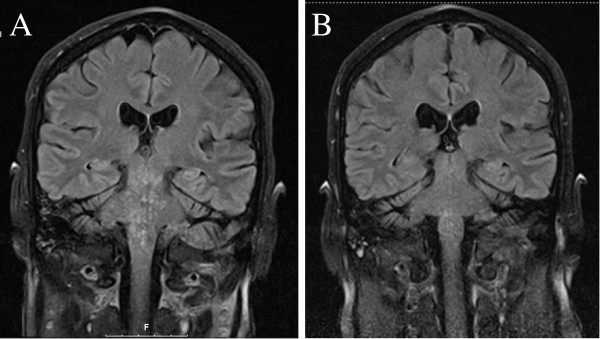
Coronal FLAIR MRI images. A. demonstrates the characteristic punctuate hyperintens lesions of the pons. B. shows diminishing of the pons-hyperintensity after glucocorticoid administration. The lesions were enhancing after gadolinium administration on T1 weighted images (not shown).
